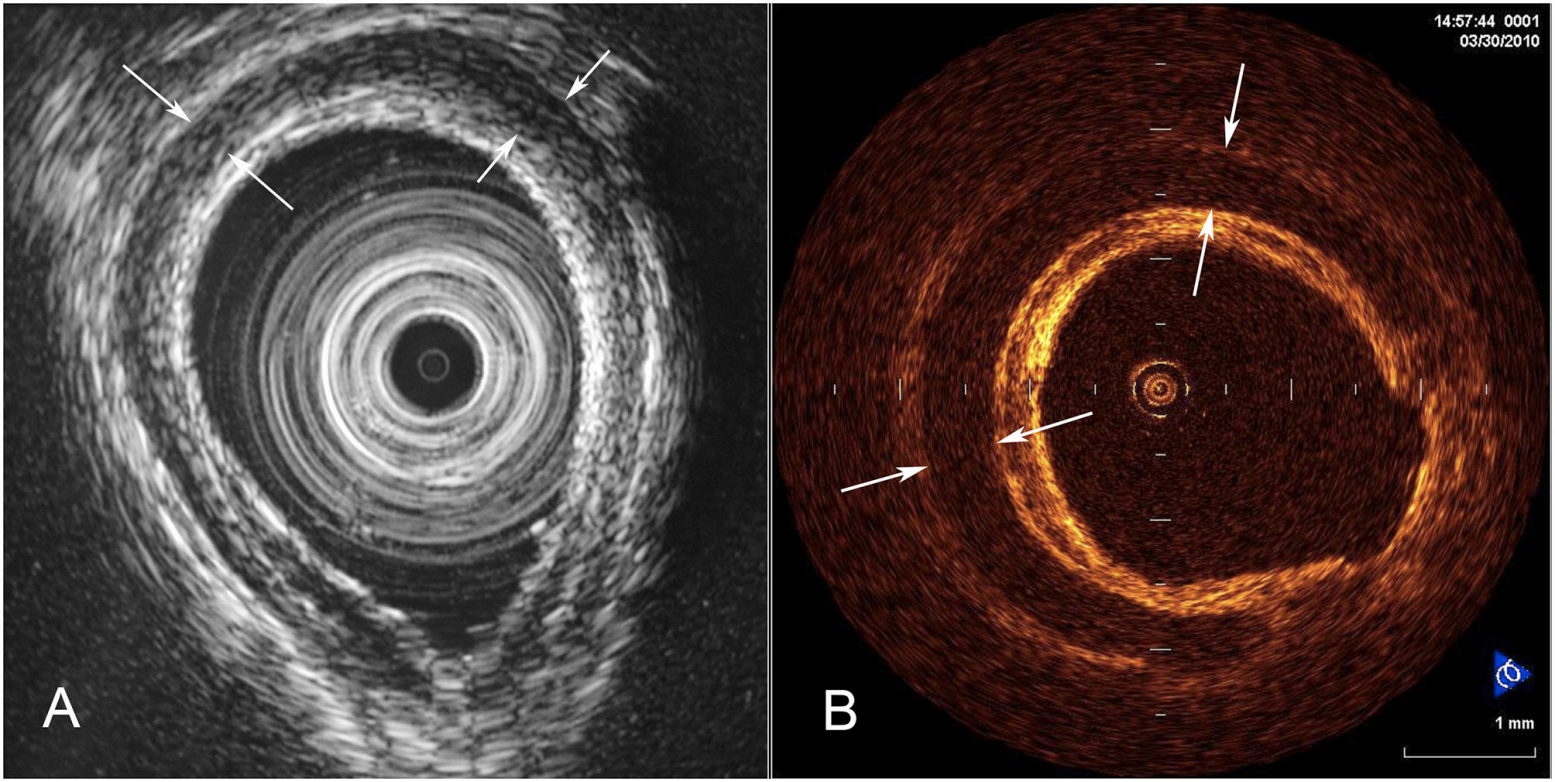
Endoluminal imaging techniques. (A) Endobronchial ultrasound image showing a cross-section of tracheal wall. The tracheal cartilage is shown as a dark (hypoechoic) band. (B) Corresponding optical coherence tomography image (from a different patient) showing the structure of the tracheal cartilage.
